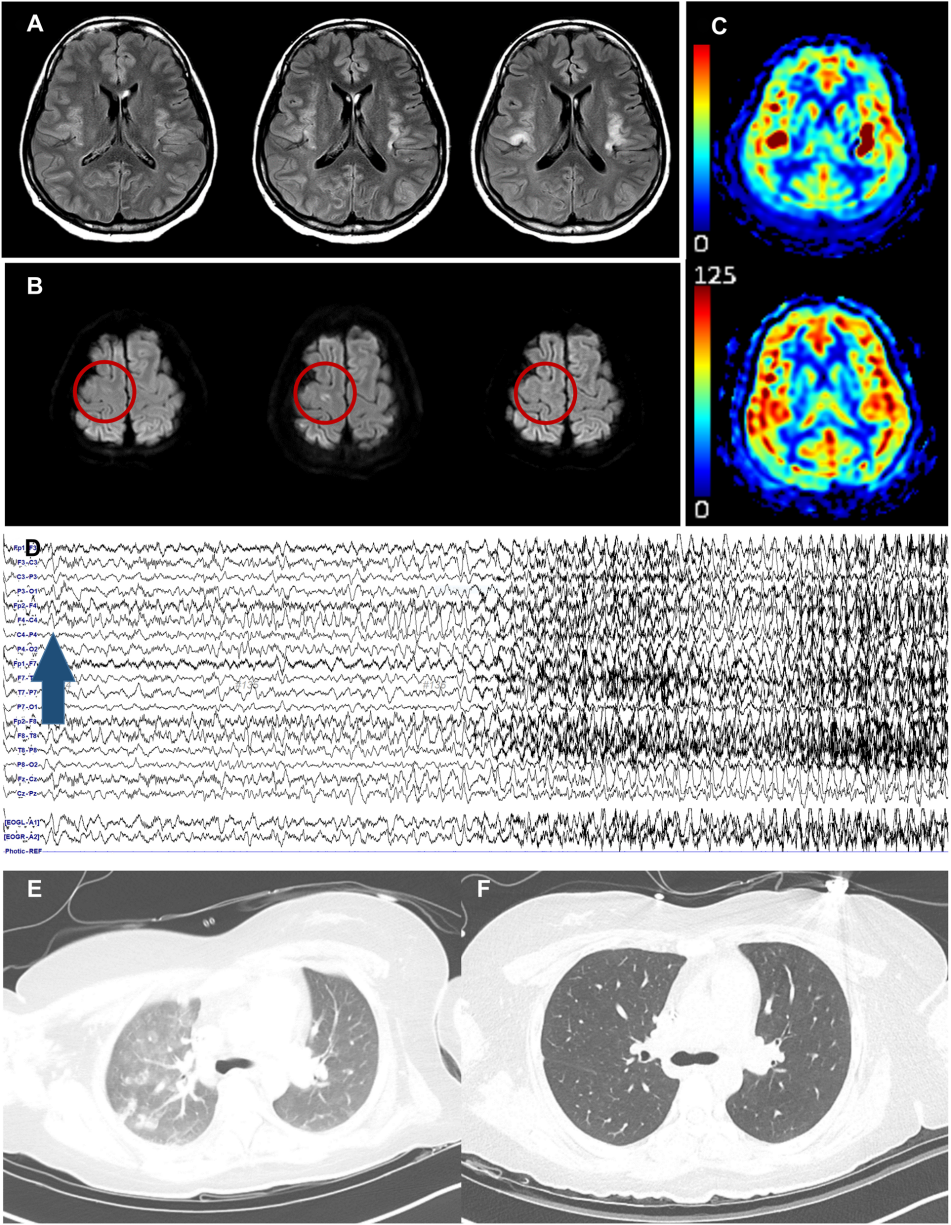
A-C are magnetic resonance imaging findings along the treatment period. A shows fluid-attenuated inversion recovery images at presentation, 7th day, and 15th day, from left to right. Note that high signal intensities involving bilateral insula intensify over time. B shows diffusion-weighted imaging at presentation, 7th day, and 15th day, from left to right. The red circle indicates the evolution of ischemic stroke lesion in the right frontal lobe, which developed on the 7th day and normalized on the 15th day. A and B show different aging stages of each lesion, supporting that the newly developed focal diffusion restriction in B is more likely due to an ischemic nature. C shows arterial spin labeling signals at presentation and two months after seizure resolution, from top to bottom. Note that the focal increase in bilateral insula, suggestive of ictal perfusion increase, is resolved. D shows the electroencephalogram findings of the patient. High amplitude polymorphic delta activities from the right frontotemporal area evolving to generalized 1-2 Hz spike-wave discharges were noted. The blue arrow indicates seizure onset from the right frontal and anterior temporal region. E is a chest computed tomography (CT) finding at presentation, which shows patchy ground-glass opacities in the right upper lung field, suggestive of COVID-19 pneumonia. F is a follow-up chest CT on day 10. Previously seen COVID-19 pneumonia is completely cleared
